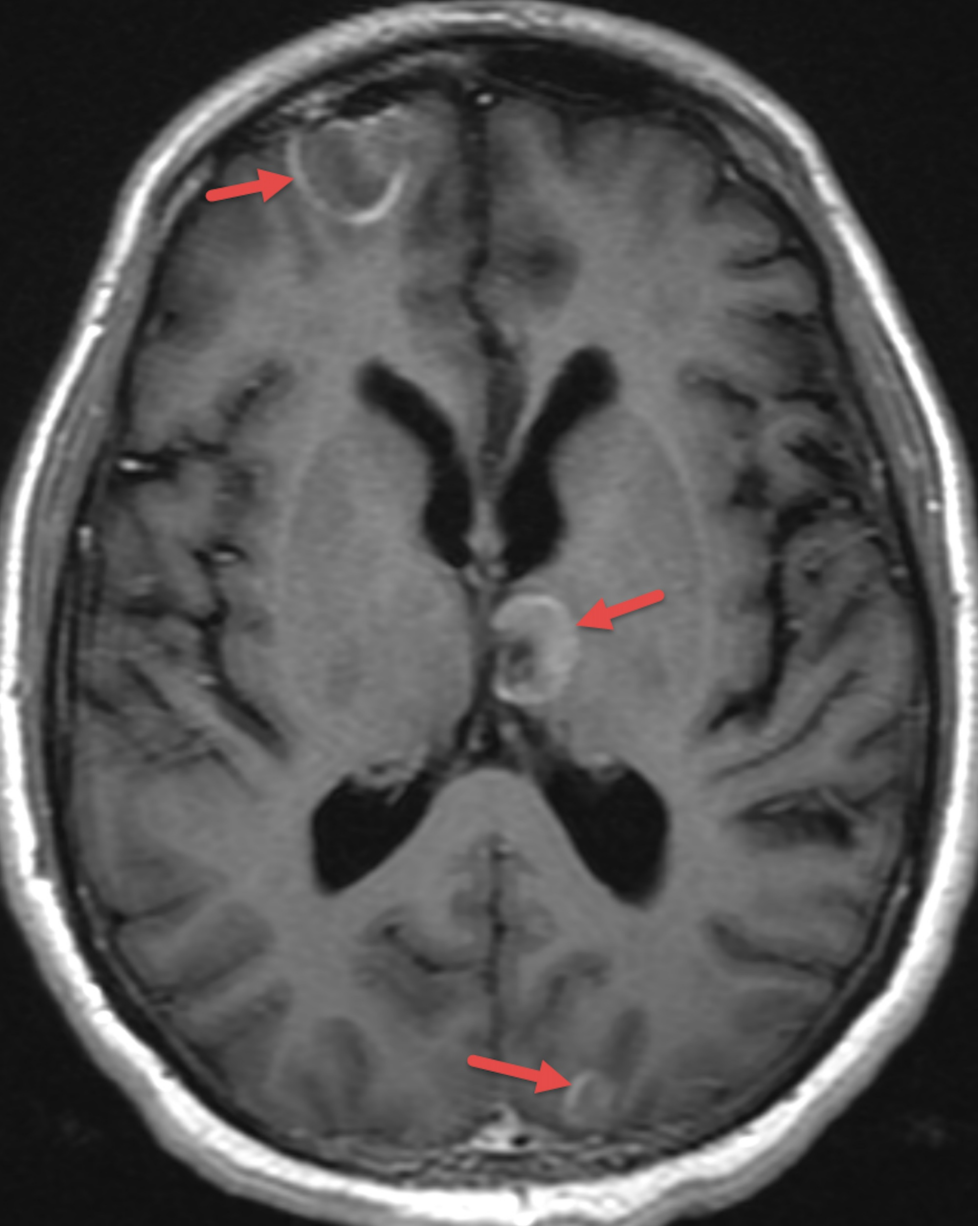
MRI of the Brain Showing Metastatic Disease
